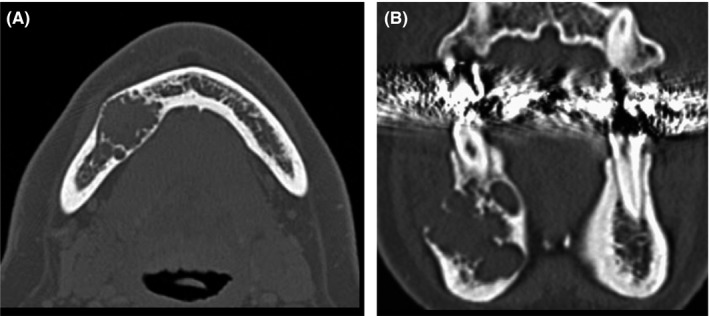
A, Preoperative computed tomographic image. Buccal and lingual expansion of the tumor is observed in the right side of the mandible. B, There is multifocal resorption in the right mandible
